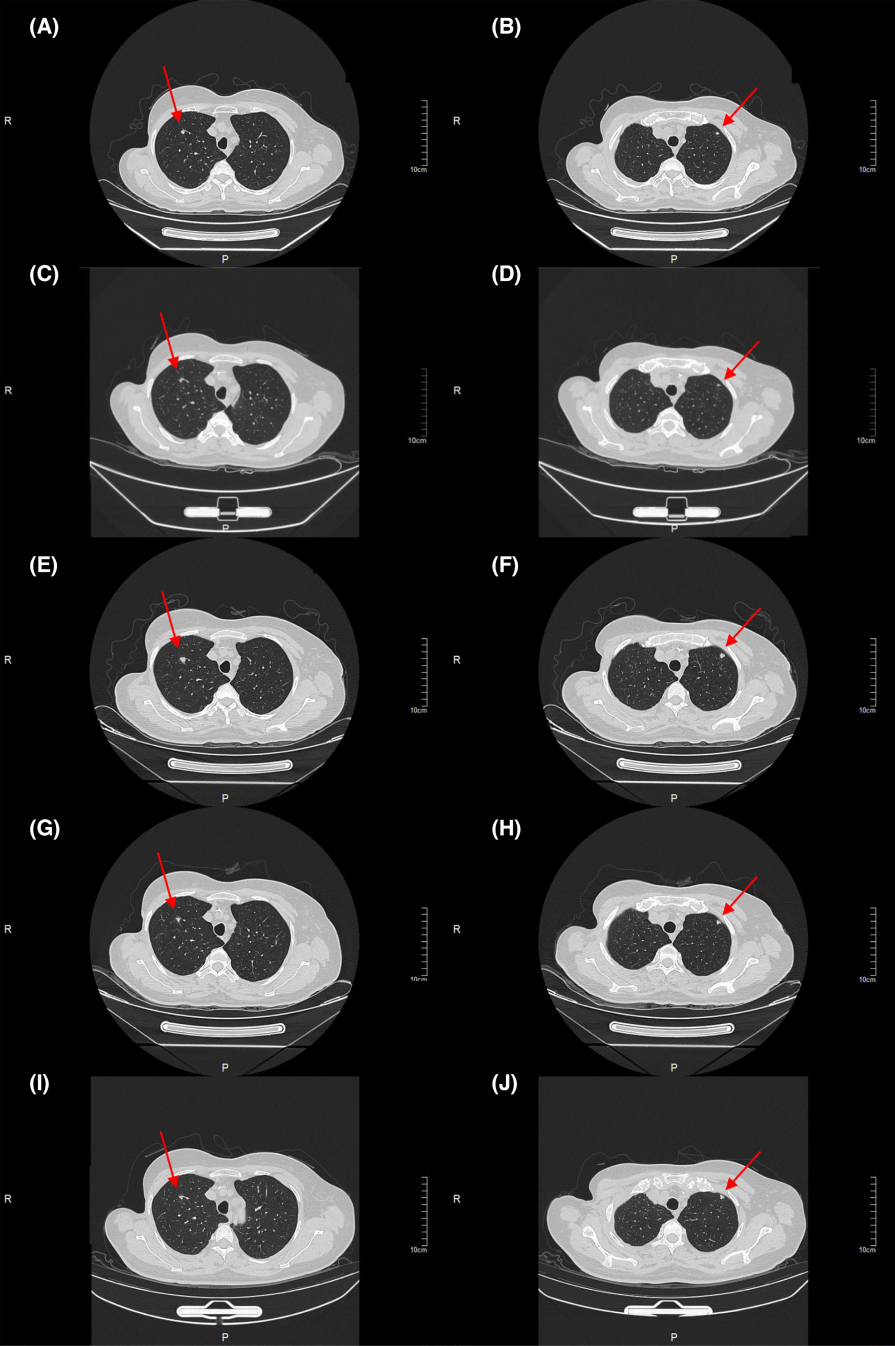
CT Image scans for lung metastases: (A, B) before nab‐paclitaxel; (C, D) after 2 cycles of nab‐paclitaxel; (E, F) after eight cycles of nab‐paclitaxel; (G, H) after two cycles of olaparib; (I, J) after four cycles of olaparib.
